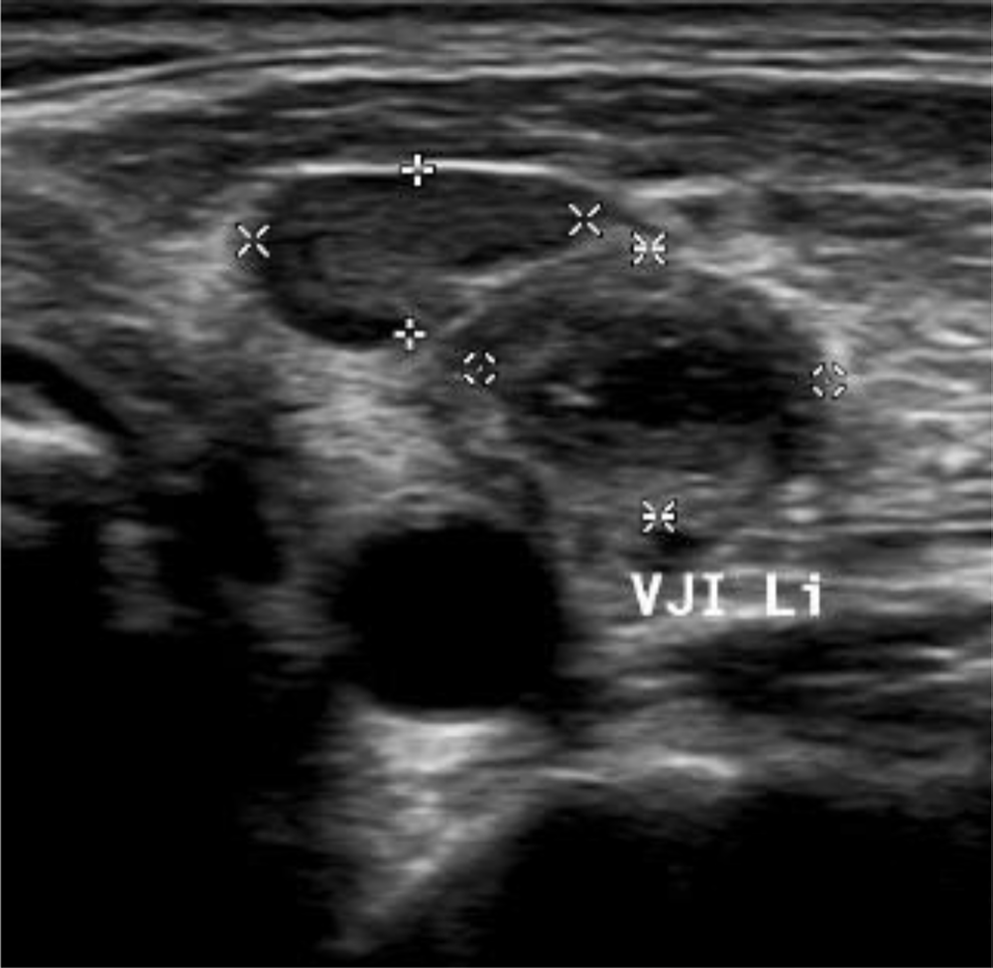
Sonography of the left neck (6 days after admission to our hospital): complete thrombosis of the internal jugular vein
